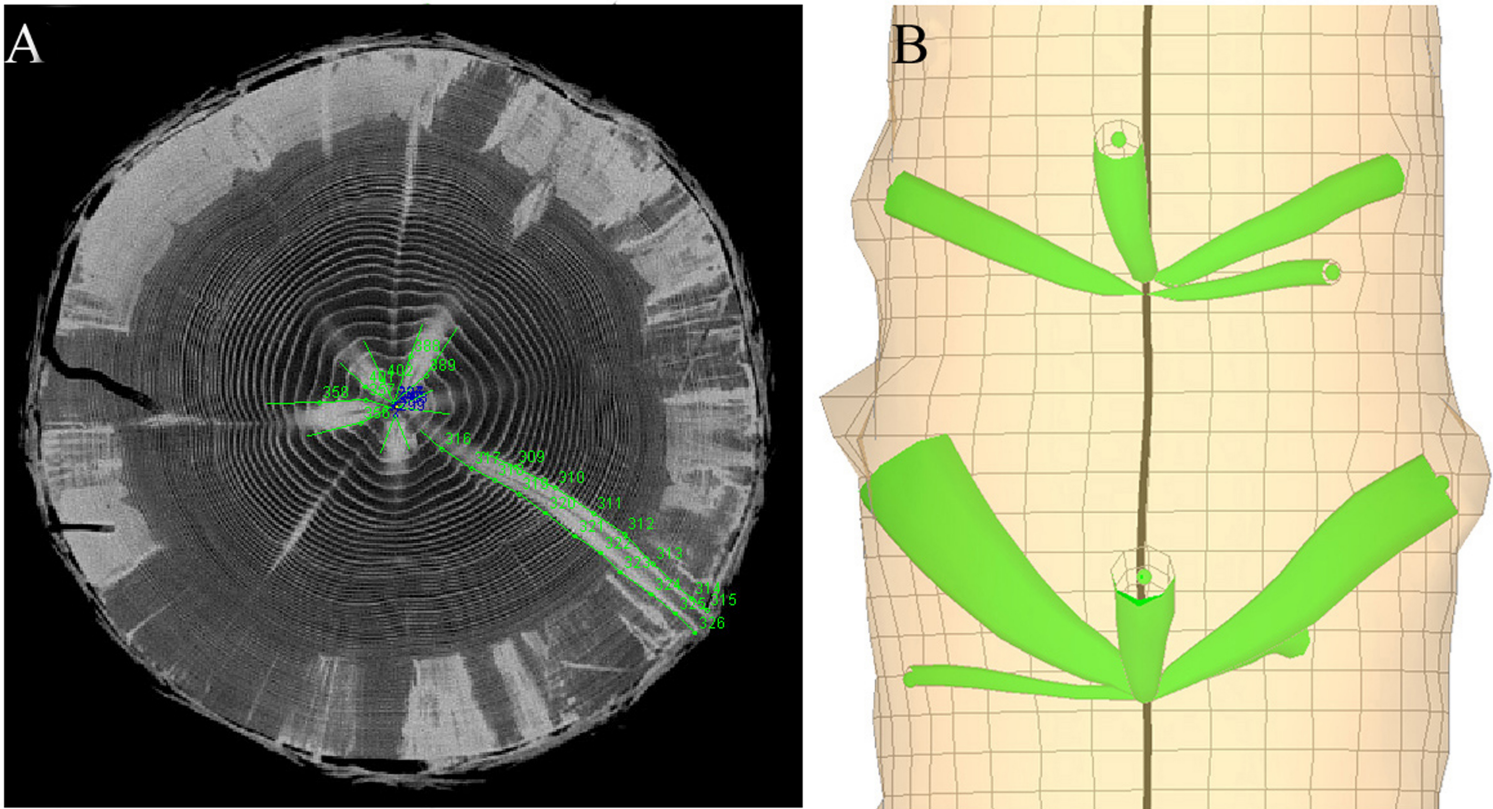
The knot extraction process. (A) Extraction of the position and diameter of each knot profile on CT scanning images using the ImageJ Java plug-in ‘Gourmand’ and (B) reconstruction of the 3D geometry of each knot using the software “Bil3D.”
